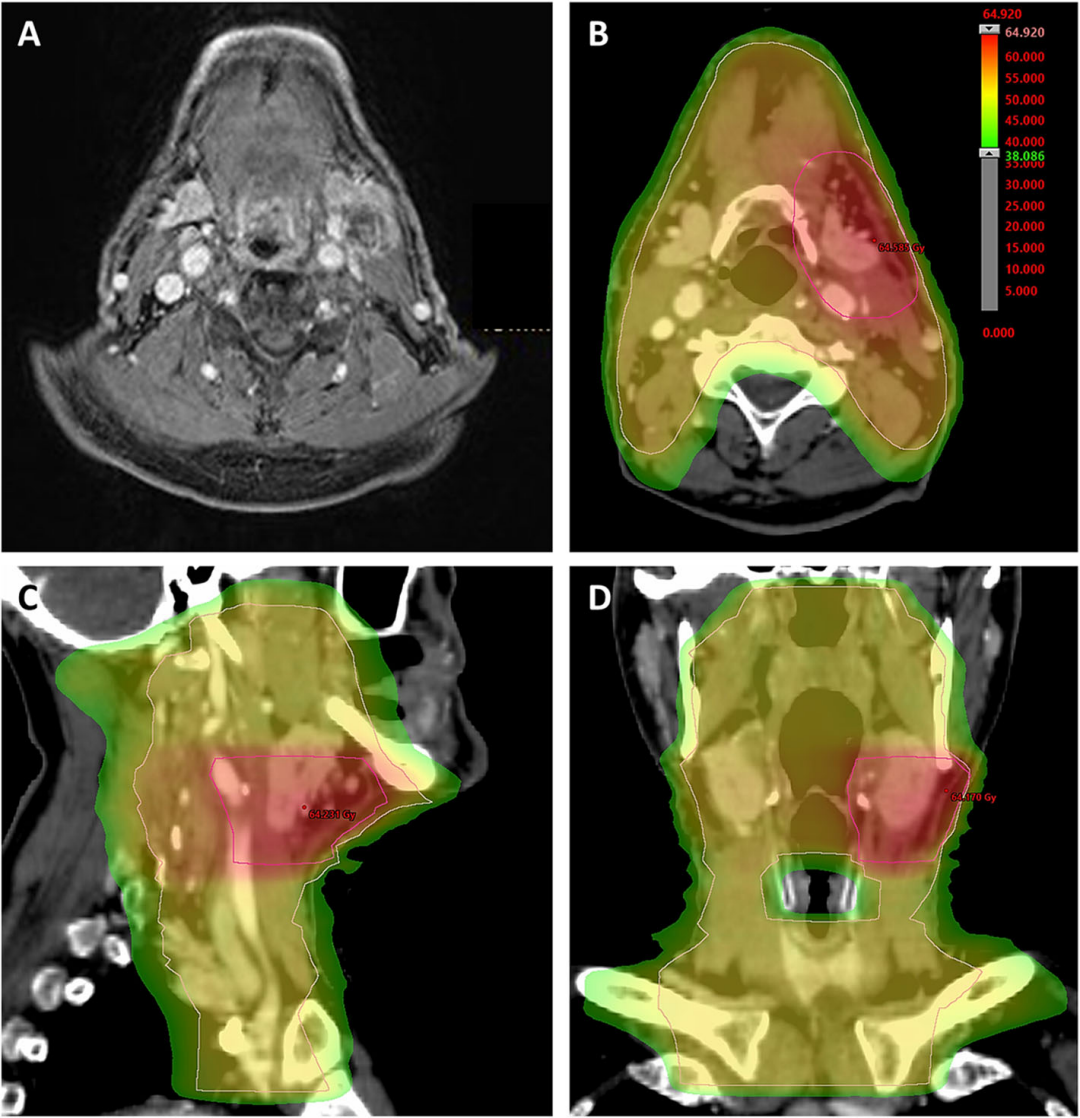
Adjuvant chemoradiotherapy for a CCUP in a 70-year-old male patient. (A) Pretherapeutic sonography, CT and MRI imaging (a) in December 2017 showed a pathological lymph node in level IIa on the left side and a suspicious lymph node in level II on the right side. A panendoscopy with multiple biopsies of different mucosal regions revealed no primary tumor. As the patient had a tonsillectomy as child, no additional tonsillectomy was performed. The recommended FDG-PET-CT was not conducted, as the costs were not covered by the patient’s health insurance. After bilateral ND in January 2018, pathological assessments showed one necrotic lymph node (1.4 cm diameter) with poorly differentiated (G3), HPV-positive squamous cell carcinoma cells in left-sided level IIa, giving a cTx pN1 cM0 CCUP according to the 7th Edition of the UICC TNM classification. Based on the recommendations of the multidisciplinary tumor board, an adjuvant cisplatin-based chemoradiotherapy with intensity-modulated radiotherapy was performed between March and April 2018. The elective lymphatic drainage and mucosa received 50 Gy in 25 fractions, while the high-risk PTV was treated with a sequential boost of 10 Gy delivered in 5 fractions. (b, c and d) Dose distribution of a volumetric modulated arc therapy plan in an axial (b), sagittal (c) and coronary (d) scan image. The last follow-up in March 2019 showed no signs of recurrence
